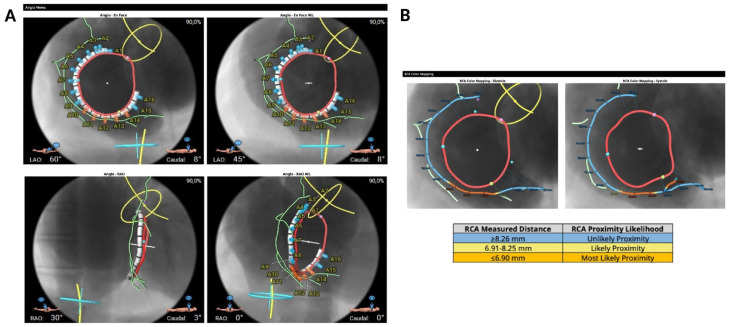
Computed tomography-based evaluation for tricuspid annuloplasty procedural planning. (A) Computed tomography-based fluoroscopic working views (face and right anterior oblique) (B) Proximity between the tricuspid annulus and the right coronary artery.
